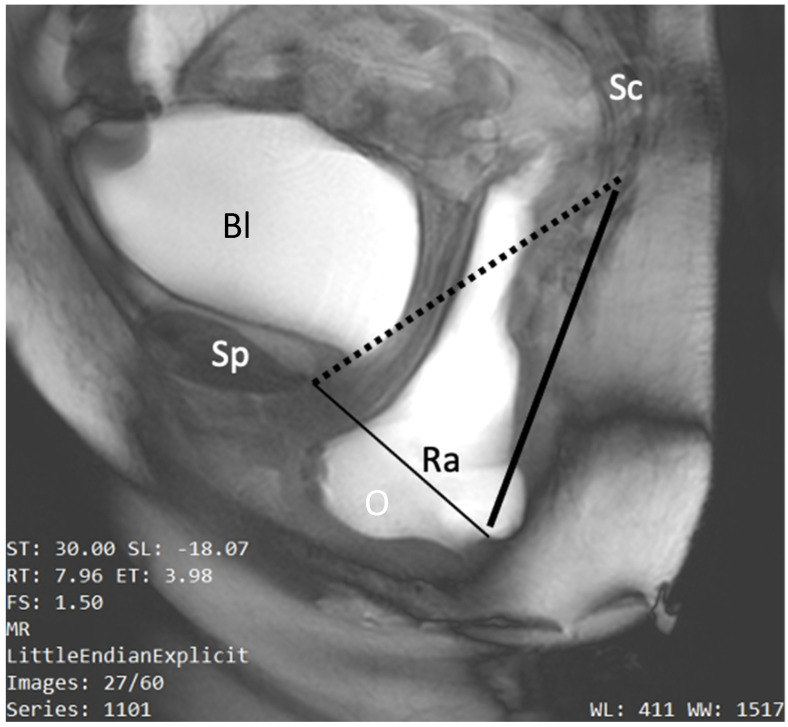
Dynamic midsagittal MR image taken during evacuation for quantification of pelvic organ descent by the HMO system according to Comiter. Pubococcygeal reference line (dotted line); H-line which measures the distance of anorectal junction to the pubic bone as index of levator hiatus width (thin continuous line); M-line indicating the length of levator plate muscle (thick continuous line). O: descent of the bladder and rectum relative to the PCL reference line; Sp: symphysis pubis–Bl: bladder; Ra: rectal ampulla; Sc: sacrococcygeal spine.
